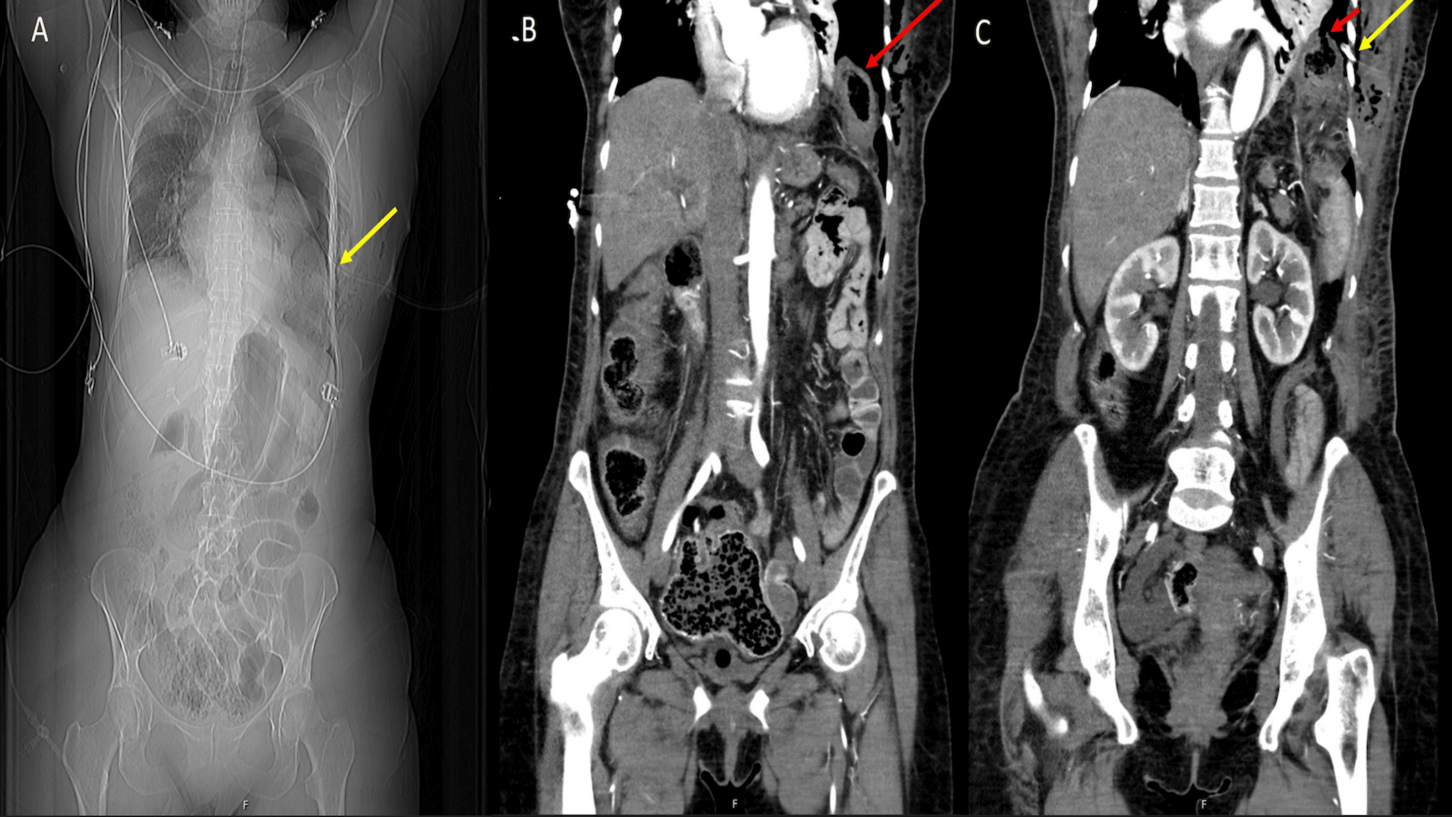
Contrast-enhanced thoraco-abdomino-pelvic CT images Contrast-enhanced thoraco-abdomino-pelvic computed tomography demonstrating the presence of the colon in the left hemithorax through a central diaphragmatic defect. Panel A - Topogram; Yellow arrow pointing to the left chest tube. Panel B - Coronal plane with red arrow pointing to the intrathoracic colon. Panel C – Coronal plane with red arrow pointing to the intrathoracic perforated colon and yellow arrow demonstrating the presence of a left chest tube.
